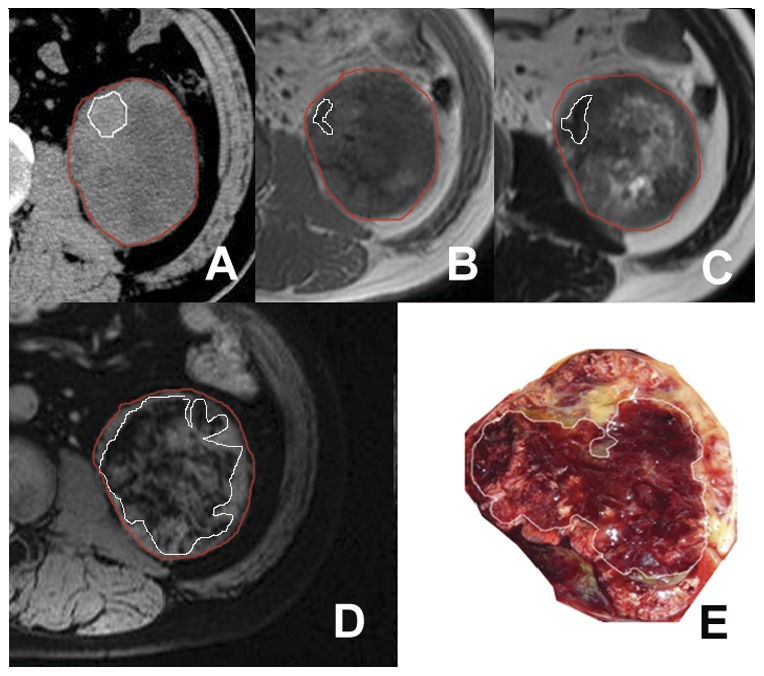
Left renal ccRCC with a grade III tumor in the Fuhrman system. In axial CT image, a patchy hyperattenuating area (area in white boundary) is seen in the tumor (area demarcated by the red boundary) (A). A hemorrhage lesion with hypointensity (area in white boundary) is seen on T1WI (B) and T2WI (C) within the tumor region (area demarcated by the red boundary). On SWI (D), an extensive hemorrhage lesion with hypointensity (area demarcated by the white boundary) is present in the tumor region. In gross pathology (E), an extensive hemorrhagic lesion (area demarcated by the white boundary) is seen in the tumor.
